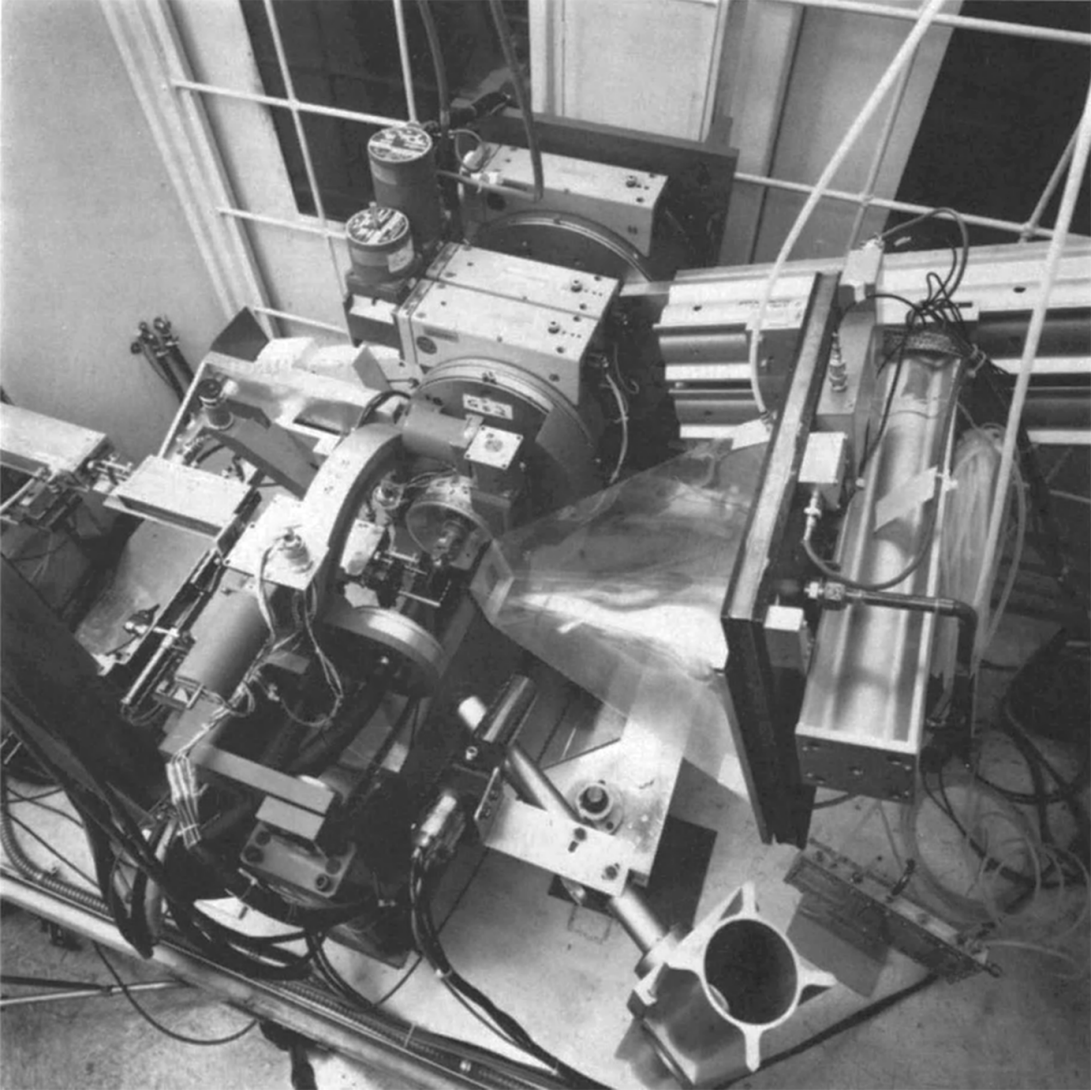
Photograph of the first MWPC area detector for MX studies (on the right, viewed from the top) and associated fast X-ray shutter, ion chambers, goniometer and other equipment installed on SSRL BL1-5 in the rear hutch *ca*. mid-1980s optimized for MX MAD data collection.
